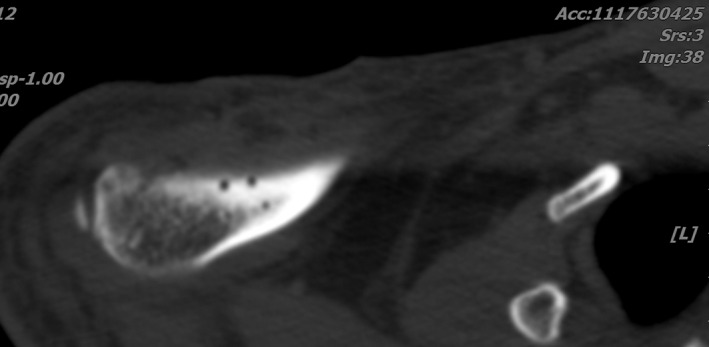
Postoperative CT‐scan shows four holes for CC ligament repair.
